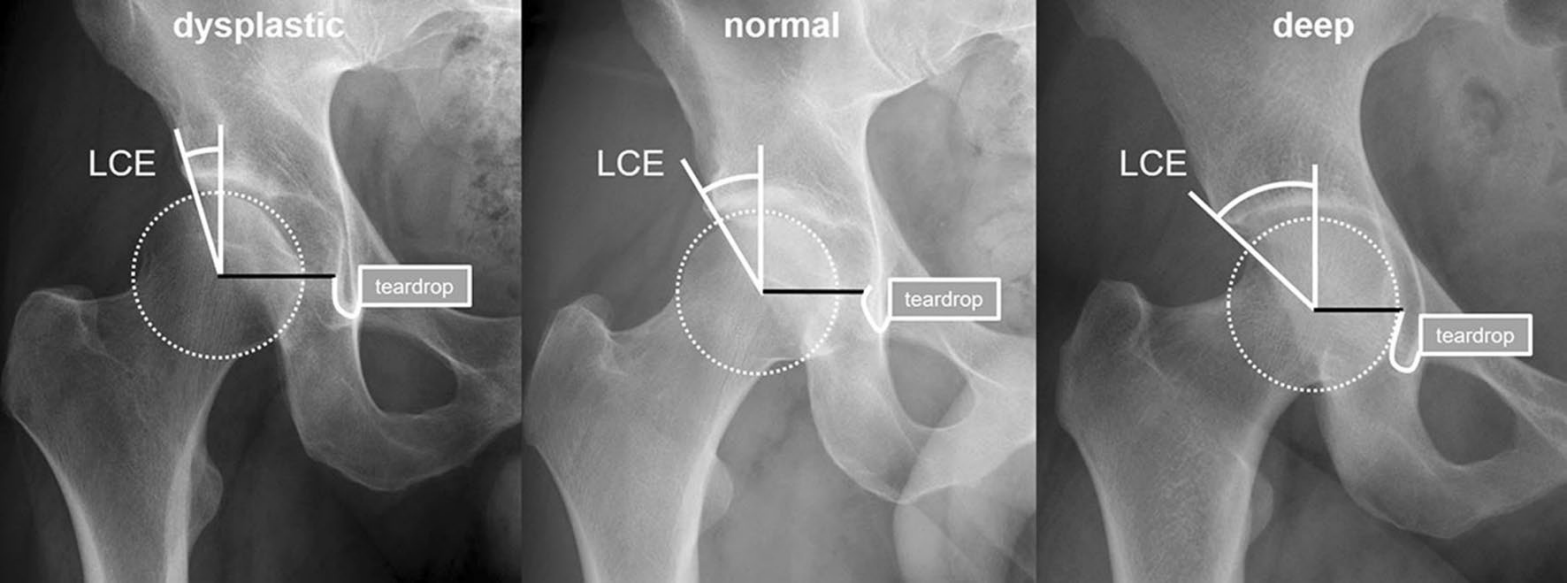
Anteroposterior radiographs of different right hip joints: the lateral center edge (LCE) angle is increasing, whilst the distance from the femoral head center to the teardrop (black line) decreases from a dysplastic to a normal and to a deep acetabular socket configuration
